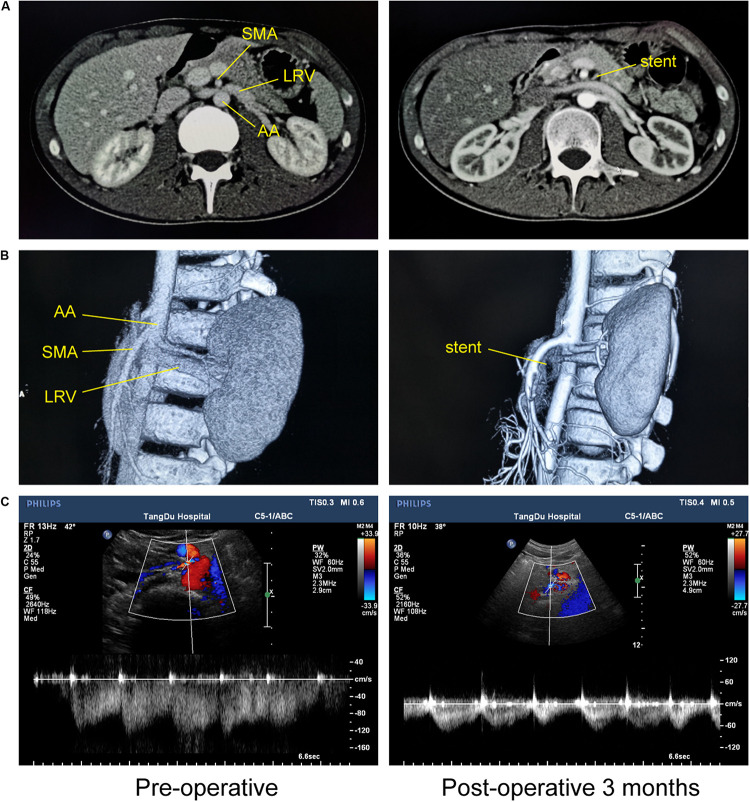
Imaging comparisons before and at 3 months after 3D-printed extravascular stenting. (A) Computed tomography. (B) 3D computed tomography reconstruction. (C) Doppler ultrasound.
